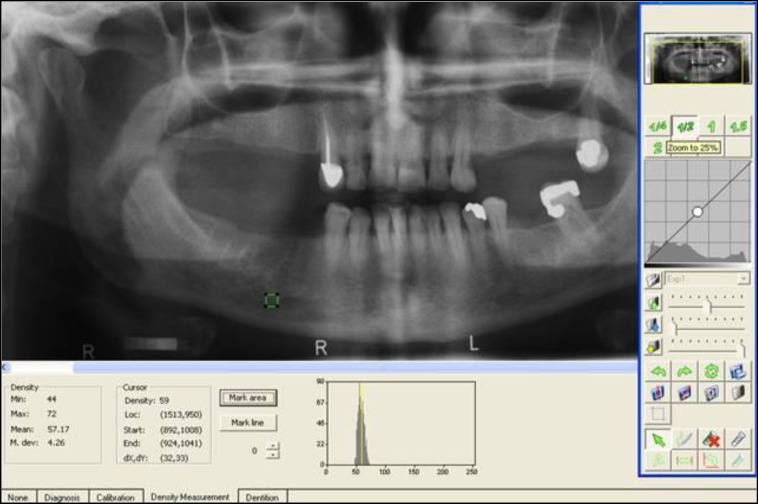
Analyzing a digital panoramic radiograph using Digora software
